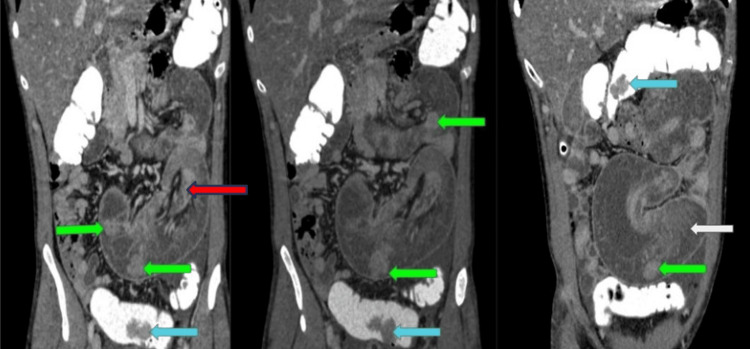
Serial coronal images of CT of the abdomen and pelvis with intravenous and rectal contrast showing mildly dilated jejunal loop (white arrow) with telescoping of jejuno-jejunal intussusception (red arrow) along with small bowel (green arrow) and large bowel (blue arrow) polyps
